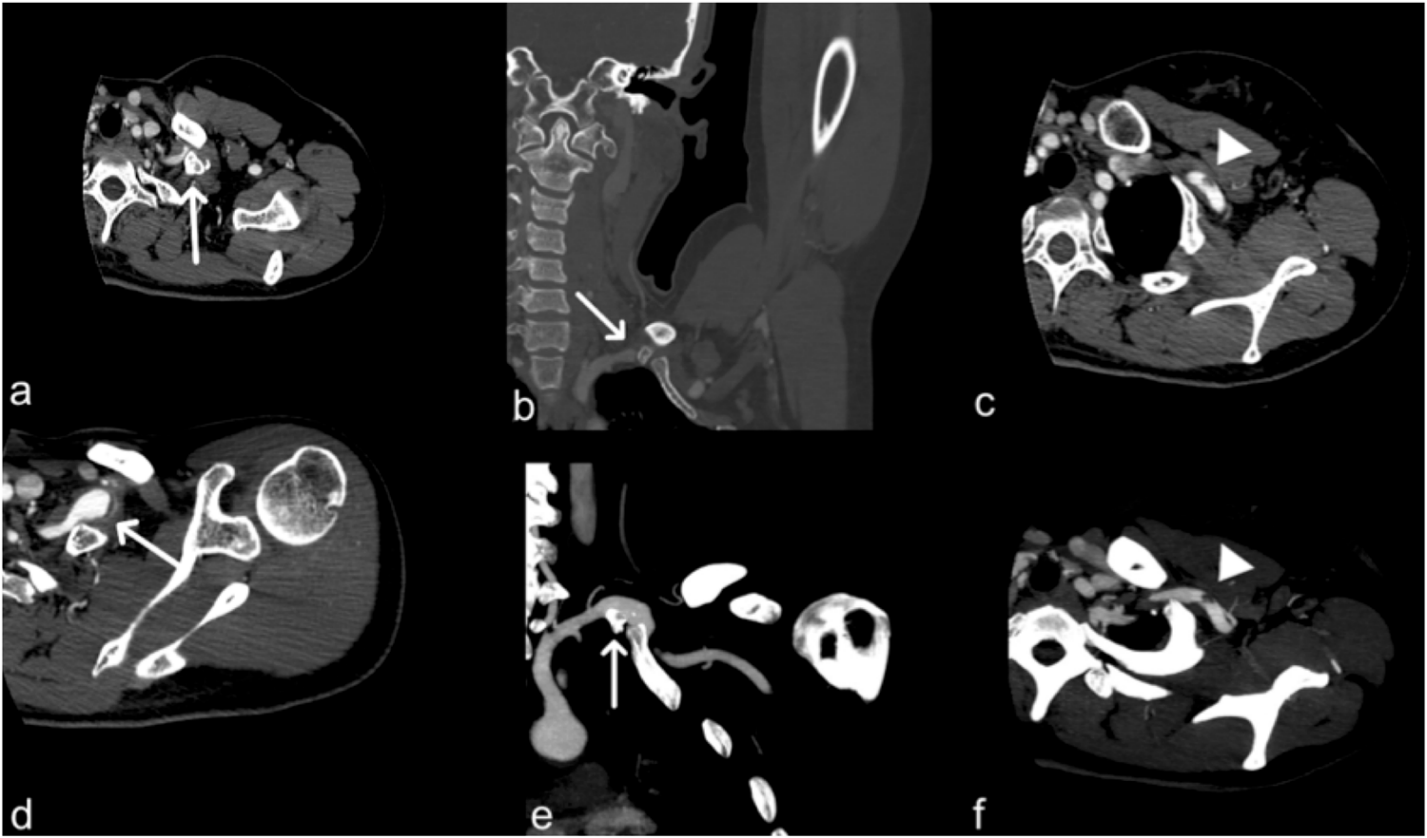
A 35-year-old female patient with a supernumerary rib. CT images after contrast medium injection, in arterial phases (a–d) and the same phase after maximum intensity projections reconstruction (e, f). They show the supernumerary rib, which causes the development of a thoracic outlet syndrome (white arrows in a—axial view and soft tissue window, and b—coronal view with bone window). c and f also demonstrate partial thrombosis of the subclavian artery (white arrowheads)
